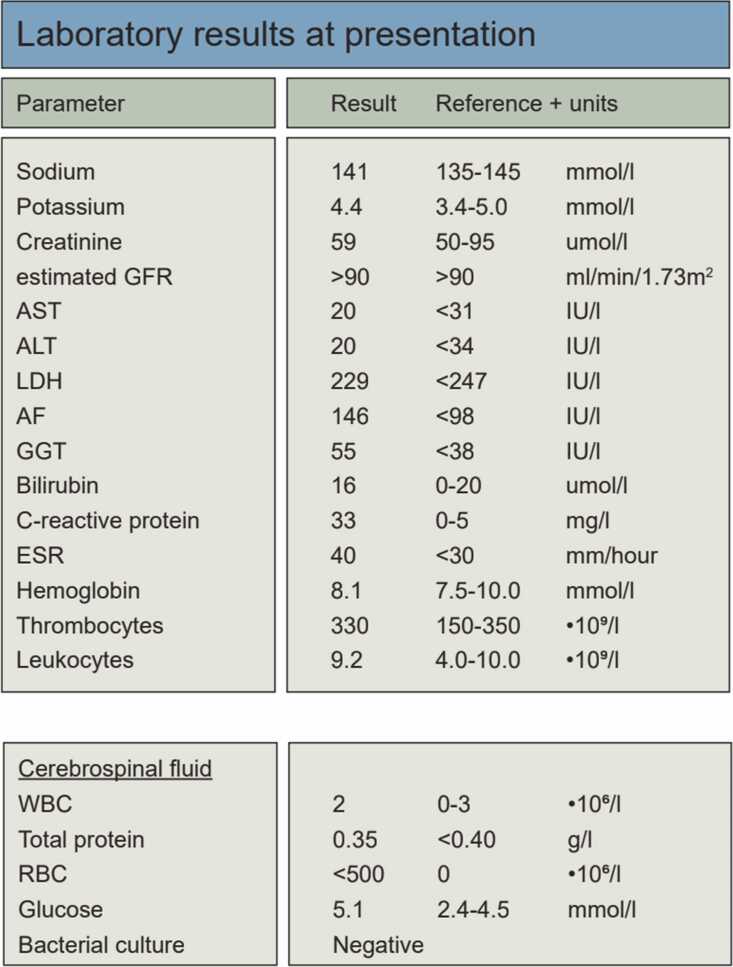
Surprisingly, further evaluation using positron emission tomography (PET-CT) and biopsy of the temporal artery showed no signs of GCA, after which the prednisone was quickly tapered and stopped. Moreover, PET-CT did not show any other signs of inflammatory/infectious activity throughout the body. After multidisciplinary reevaluation, we concluded that the most likely diagnosis is a tertiary stage ocular infection of syphilis, also known as syphilitic uveitis. She was subsequently treated with high-dose intravenous benzyl penicillin (24million units/24 h) during two weeks. A few weeks later, she reported markedly improved vision, with eventually no residual vision loss.
